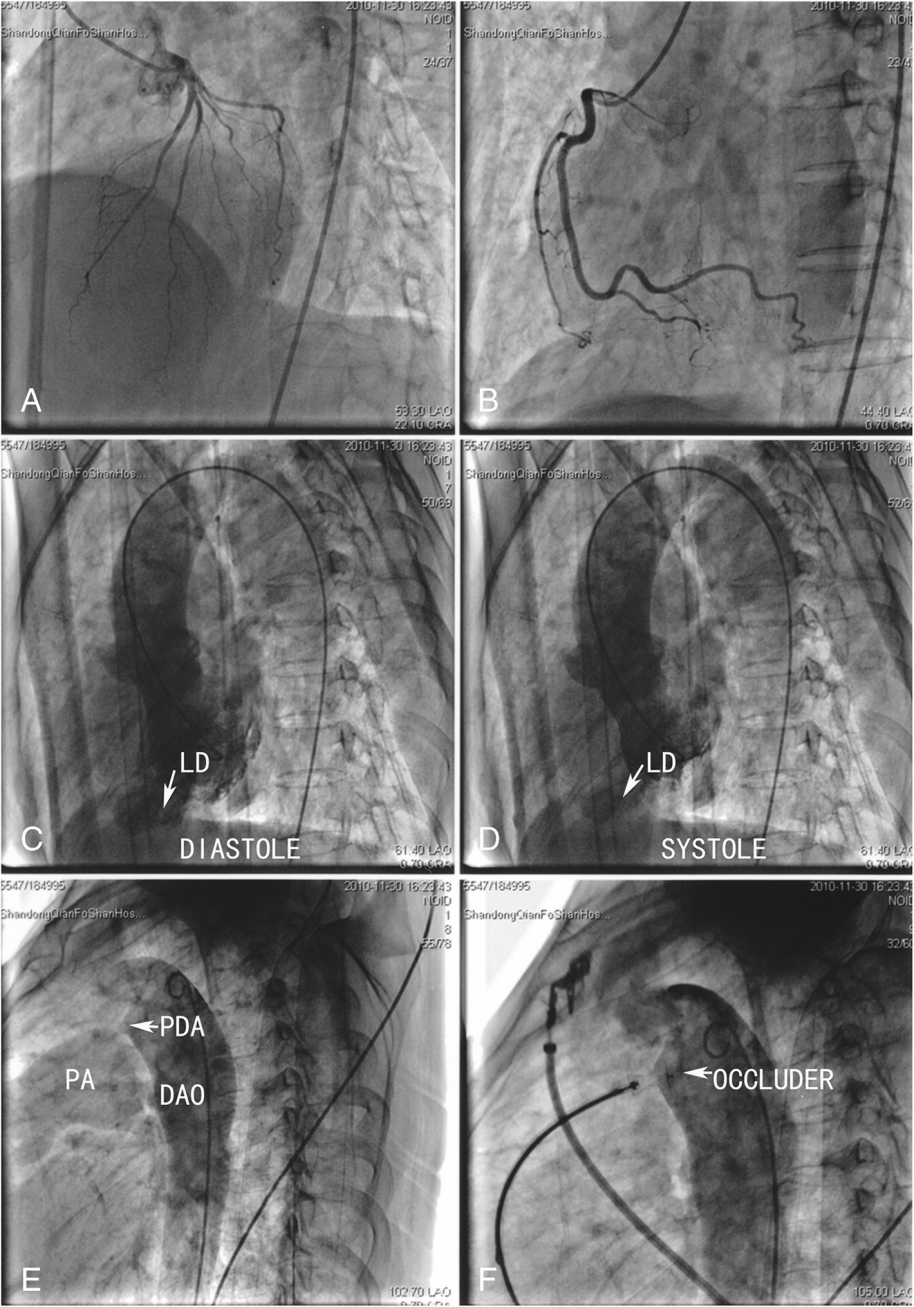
Coronary angiography showing a normal coronary artery (a and b); Left ventriculography of a left anterior oblique view showing an LD (c and d) of similar shape compared with the Helical CT (Fig. 3d); and an aortic angiogram showing a residual PDA shunt to the pulmonary artery (PA) and good position of the PDA occluder (e and f)
